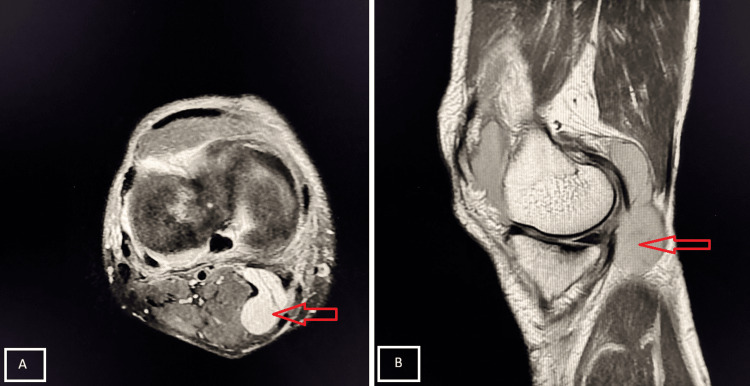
Baker’s cyst in the popliteal fossa Axial PD-FS (A) and sagittal (B) T1-weighted MRI demonstrate a well-defined cystic lesion with internal septations located between the semimembranosus tendon and medial head of the gastrocnemius, communicating with the knee joint via a narrow neck, consistent with Baker’s cyst (red arrows). PD-FS, proton density fat-suppressed
